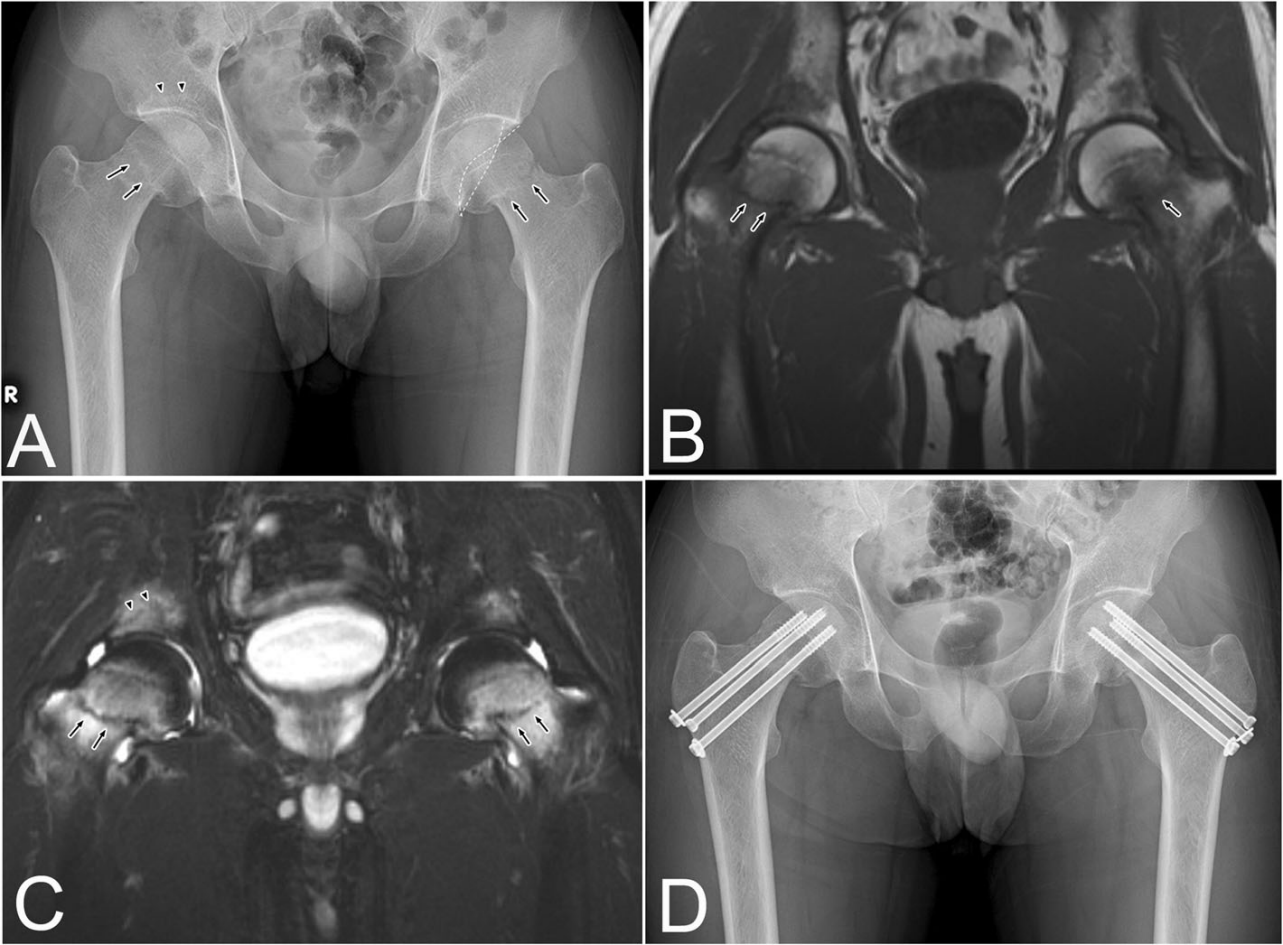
a Initial radiograph showing bilateral femoral neck fracture (arrows). The osteosclerotic lesion was observed at the right acetabulum (arrowheads). In the left hip, the cross-over sign was seen (dotted lines). Coronal T1-weighted image (repetition time/echo time [TR/TE] = 474/14 msec) showing diffuse low-signal intensity in the femoral head and lower neck (b) and the corresponding high-signal intensity on the T2 fat-saturated image (TR/TE = 3500/87) (c). Coronal T1 (b) and T2 fat-saturated (c) images revealed the fracture line in the bilateral femoral neck (arrows) and in the right subchondral region of the acetabulum (c) (arrowheads). d Bilateral osteosyntheses using multiple pinning method were simultaneously performed in the bilateral hips
